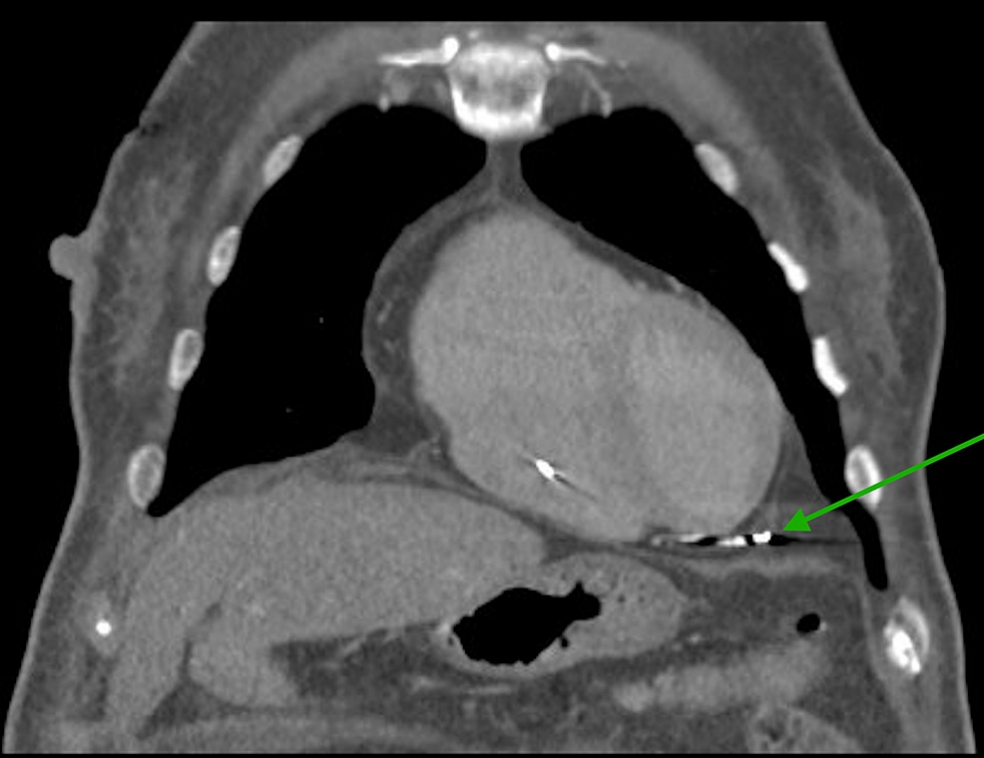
CT scan of the chest, coronal slice, showing the RV lead beyond the ventricular wall RV: right ventricular
